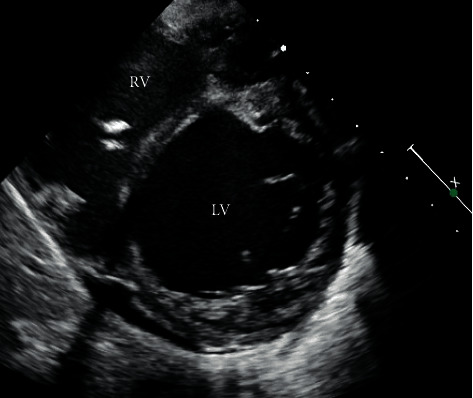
Parasternal short-axis view of the left ventricle revealing hypertrabeculations and deep intertrabecular recesses.
